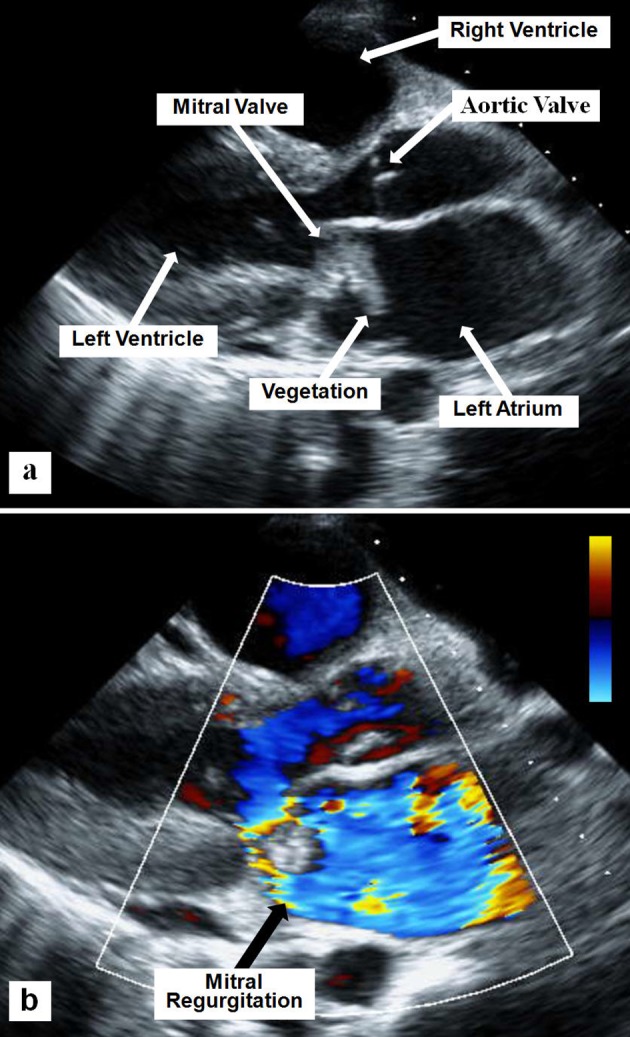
Two-D parasternal long axis echocardiographic view showing the mitral valve vegetation (a) and the severe mitral regurgitation using superimposed color flow Doppler (b).
